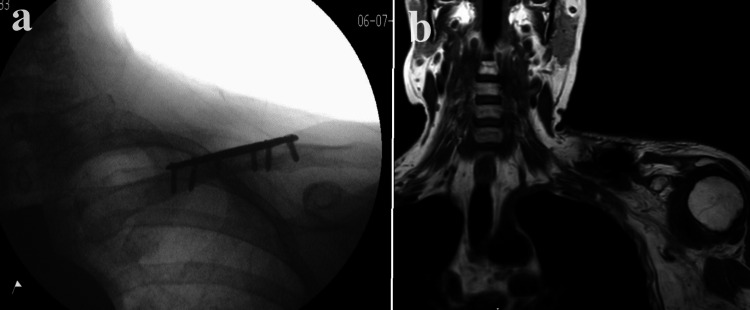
Case 3: a displaying intra-operative fluoroscopic evaluation, indicating satisfactory reduction, and b showing post-operative MRI, revealing the plexus appearing in continuity
